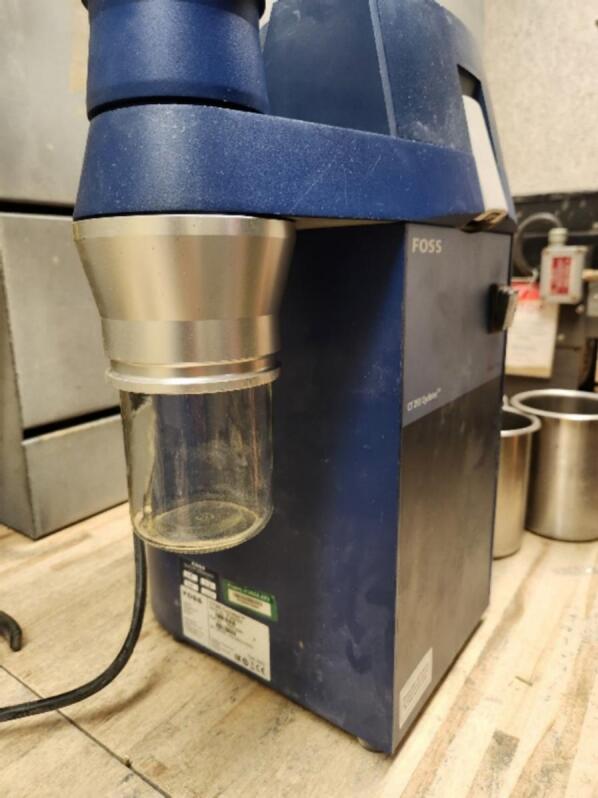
Foss CT 293 Cyclotec grinder with the glass sample jar installed. The 350 ml jar screws into machine and ground samples drop directly collected into the container. However, the sample needs to be transferred into another storage container before grinding the next sample.
